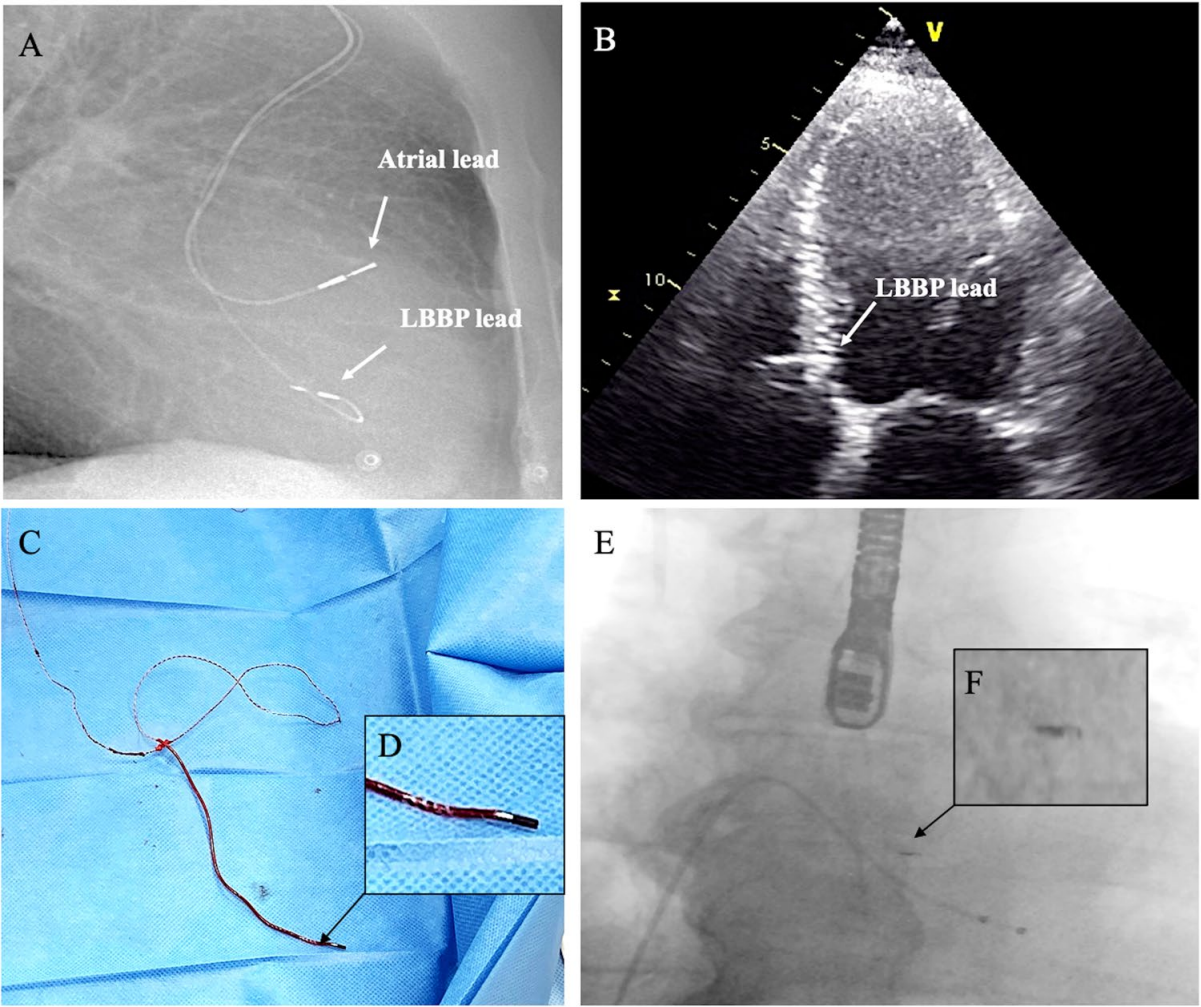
Patient no. 6. Lateral chest X-ray view showing the atrial lead and the LBBP lead (A). Four-chamber echocardiography view showing the LBBP lead deep into the interventricular septum (B). Removed 3830 lead (C). Note the absence of the screw after lead extraction (D). Fluoroscopy view immediately after removal of the leads under TE guidance (E) showing the retention of the screw into the interventricular septum (F). LBBP, left bundle branch pacing
